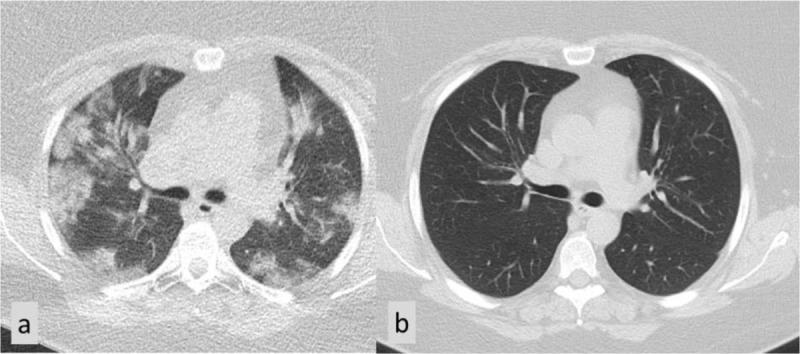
A 43-year-old woman showed on covid-scan multiple areas of ground-glass opacities in both lungs (a). A control CT scan after 18 weeks could not show any abnormalities anymore (b).
